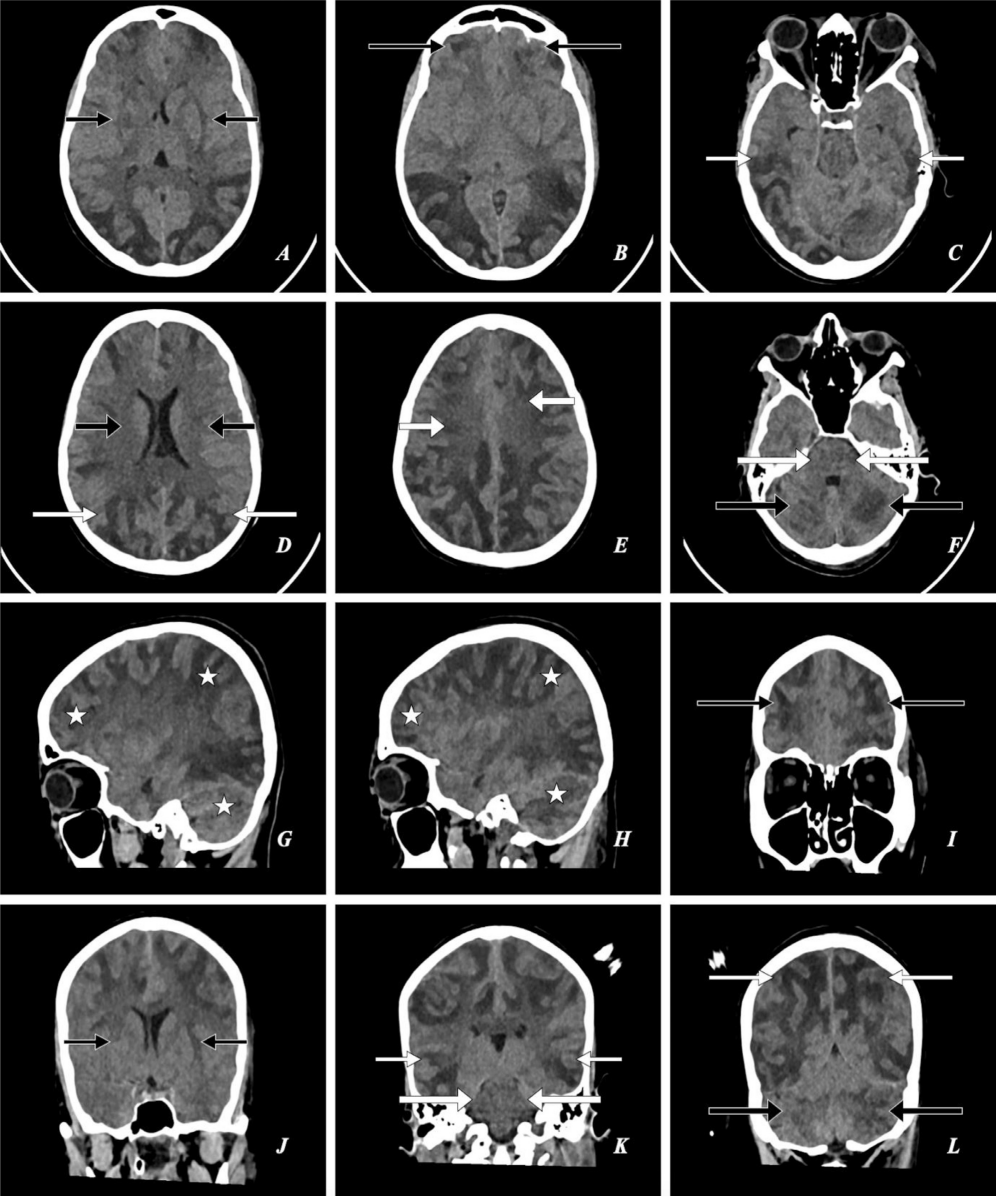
A 23-year-old female presented to the emergency department with complaints of a series of 4 seizures accompanied by severe headaches, visual disturbances, and altered mental status. *Follow-up (3 days) Computed Tomography (CT)—Brain: (A, B, C, D, E, F) Axial, (G) Right Para-Sagittal, (H) Left Para-Sagittal, (I, J, K, L) Coronal* shows multifocal/multi-centric asymmetrical hypodensities (vasogenic edema) in the bilateral ganglio-capsular regions, bilateral thalami *(short thin black arrows),* bilateral frontal lobes *(large thin black arrows),* bilateral temporal lobes *(short thin white arrows),* bilateral parieto-occipital lobes *(large thin white arrows),* bilateral corona radiata *(short thick black arrows),* bilateral centrum semiovale *(short thick white arrows),* bilateral hemipons and bilateral middle cerebellar peduncles *(large thick white arrows),* bilateral cerebellar hemispheres *(large thick black arrows).* Vasogenic edema in the deep gray matter, deep white matter, sub-cortical white matter U-fibers and cortical white matter *(white asterisks) [Significant reduction / resolution of Vasogenic edema].*
